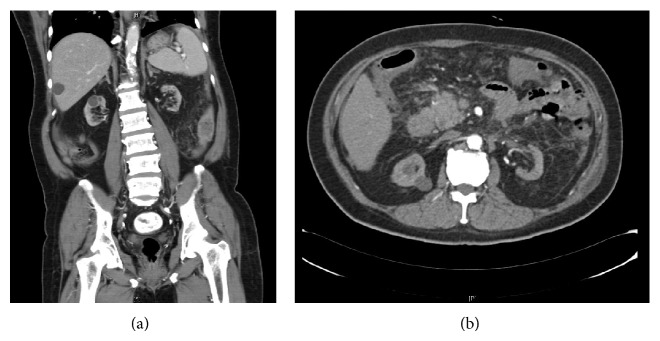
CT findings showing a 1.3 × 1.3 cm enhancing lesion at the upper pole of right kidney.
